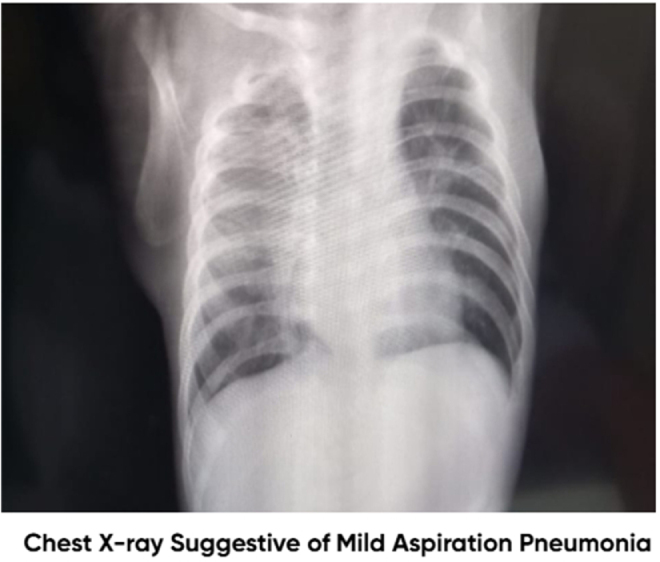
Chest X-ray demonstrating bilateral pulmonary infiltrates.
